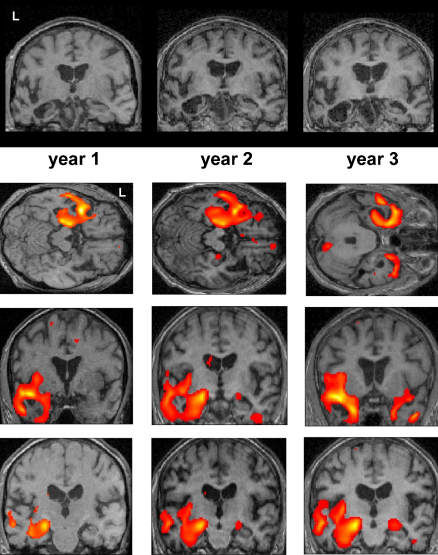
Structural MRI brain scans of the patient. Top panels show coronal sections through the brain of patient AM at the level of the mid-temporal lobe for each year. Running vertically below each year's coronal section are further axial and coronal sections from that year's MRI scan superimposed on which are the results of the VBM analysis for that year. The pronounced atrophy of cortical and medial left temporal regions is apparent at year 1. Scans from the subsequent years show a progression in this atrophy, and additional pathology starting in homologous areas on the right. See main text, and Table S1 (in Supplementary Materials) for full details of the VBM findings.
